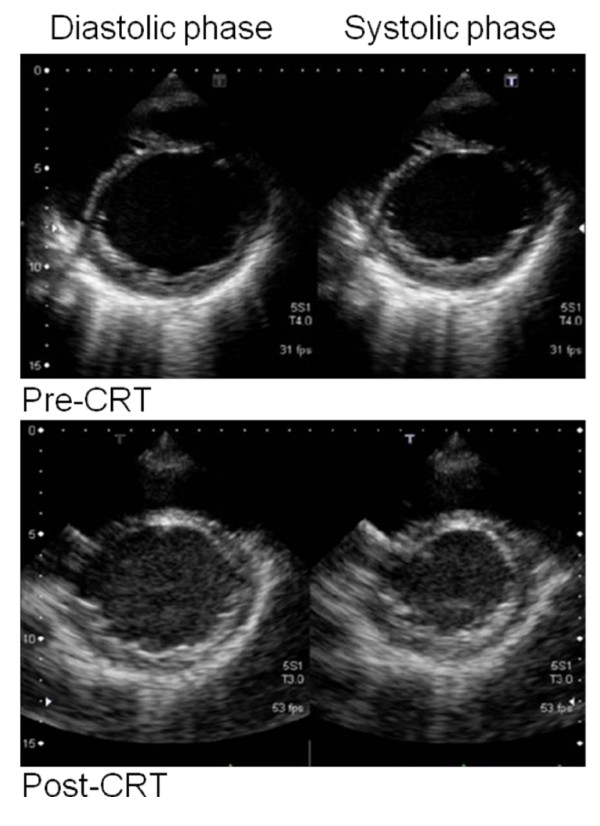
Echocardiogram before and after the CRT placement. Each panel shows the short axis view of the LV cavity. Upper panels: The LVDd was 59.3 mm and LVEF was 31.2% before the CRT placement. Lower panels: The LVDd was 61.3 mm and LVEF was 51.3% after the CRT placement.
